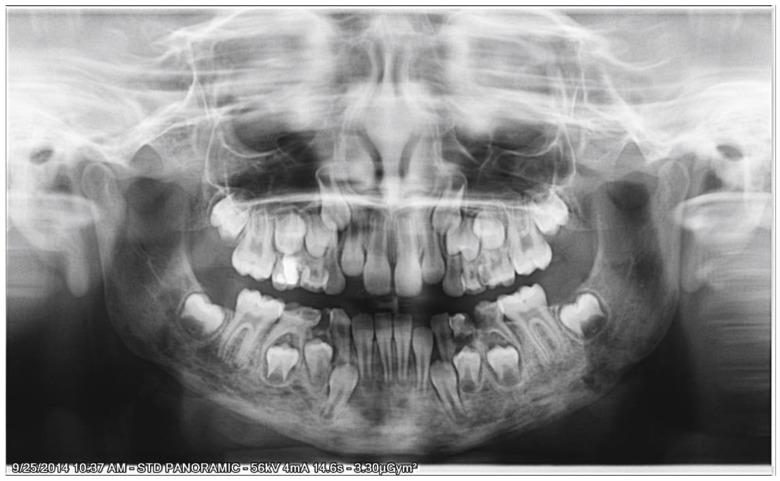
Panoramic radiograph showing the “onion-skin” appearance of the bone at full length of the mandible.
